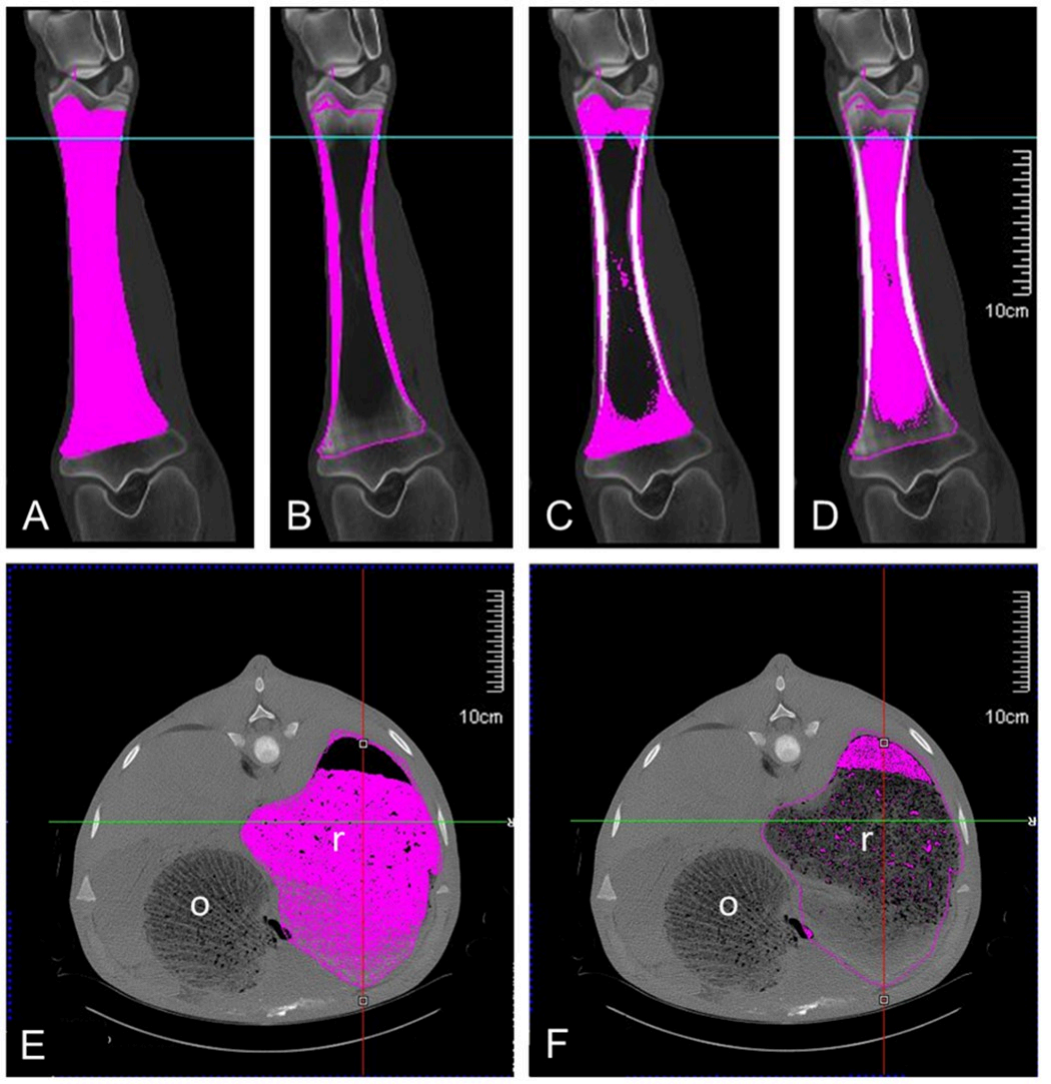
CT scan images of a tibia and a rumen. Photographs showing the different parts of a tibia discriminated by a CT scan with automatic thresholding (A, total bone; B, cortical bone; C, spongy cancellous bone; D, medullary cavity) and of a rumen (E, bolus; F, air) obtained by x-ray CT scanning. The bar represents 10 cm. r, rumen; o, omasum.
